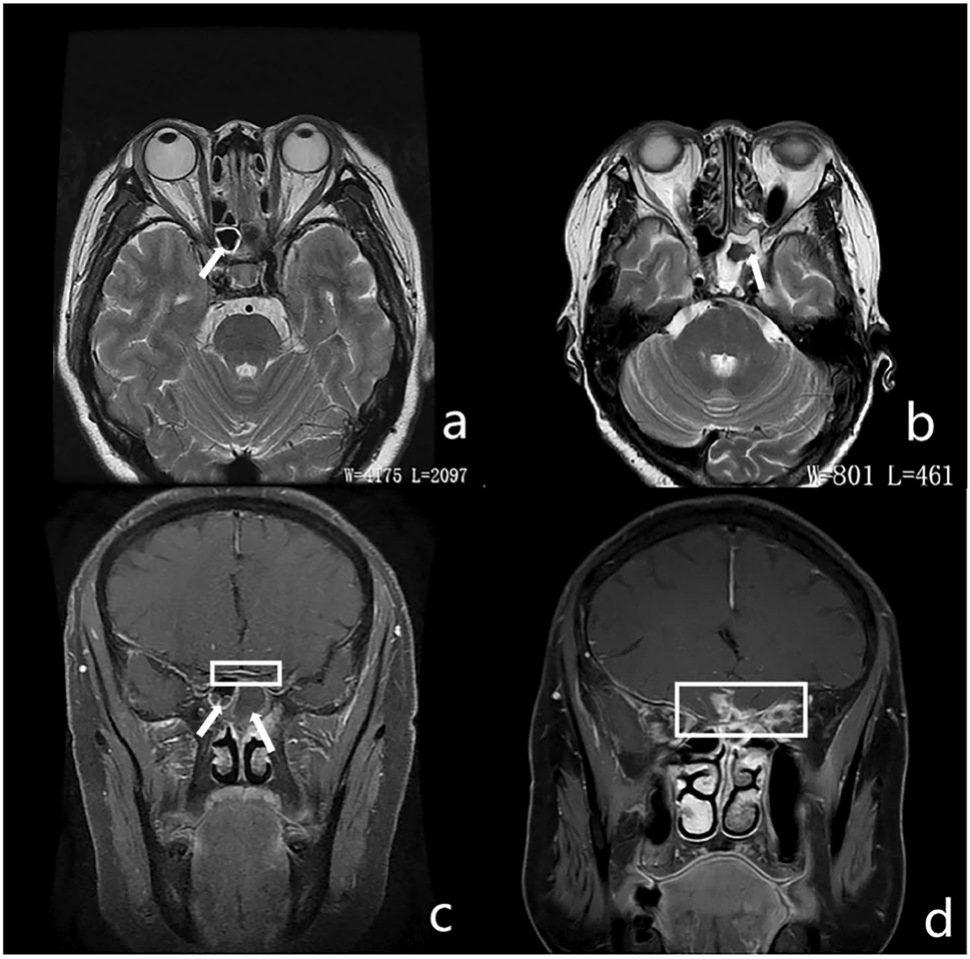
MRI findings of FSS. (a, b) show low signal of right sphenoid sinus on T2WI (white arrow), peripheral mucosal thickening and linear enhancement (white arrow). Irregular low T2 signal shadows can be seen in the left sphenoid sinus (white arrow). (c, d) show the hypointensity in bilateral sphenoid sinus on T1WI , without enhancement (white arrow). The lesion invades the skull base, and enhancement can be seen in the adjacent skull base membrane (white box).
